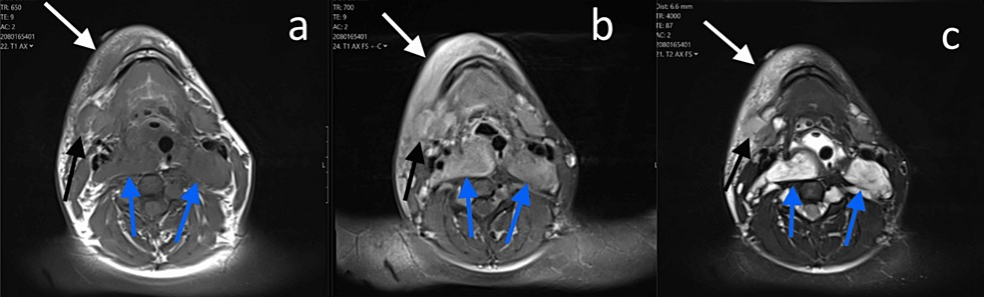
MRI of neck's soft tissues T1-weighted (a), T1-weighted postcontrast (b), and T2-weighted (c) sequences. White arrows: extensive edema and enhancement of the subcutaneous tissues along the right lower lip.
